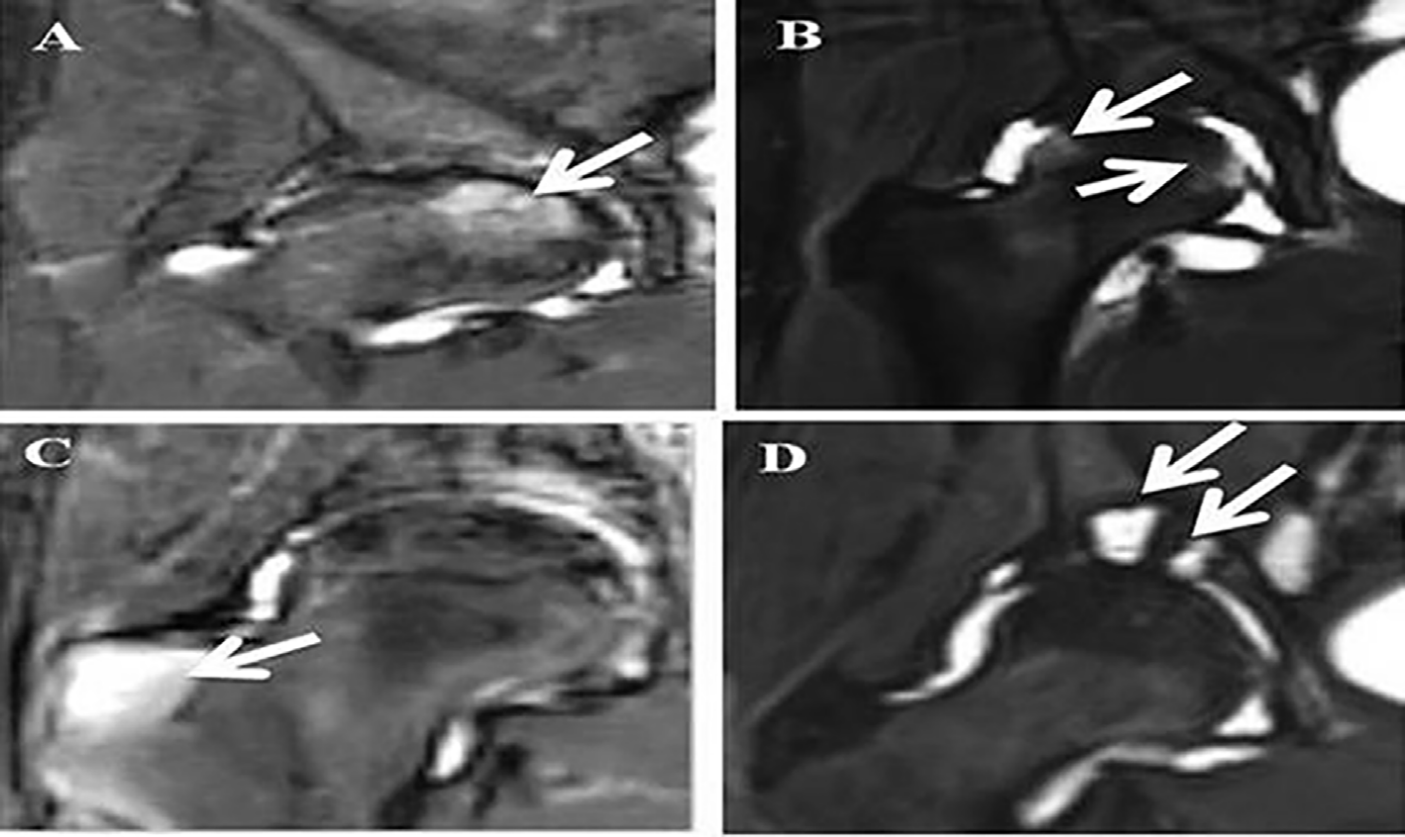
MRI STIR images of hips in AS patients. Legends: (A) White arrow points at a deep BME lesion in the femoral head; (B) White arrows point at superficial BME lesions in the femoral head; (C) White arrow points at a BME lesion in the greater trochanter; (D) White arrow points at two cysts in the acetabulum.
